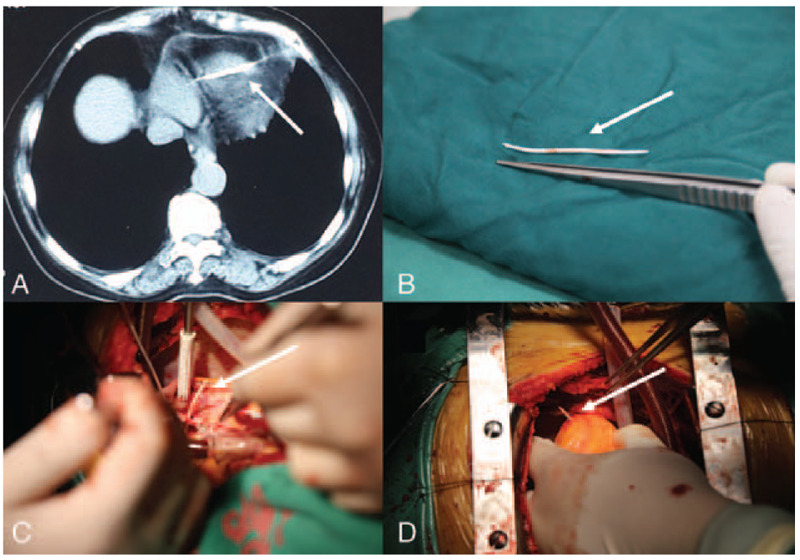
(A) Chest computed tomography showing a high-density shadow extending from the bottom of the heart to the anterior chest wall (indicated by arrow). (B) Foreign body after removal with forceps for scale. (C) Foreign body piercing the posterior flap of the tricuspid valve (indicated by the arrows). (D) Foreign body penetrating the anterior wall of the right ventricle (indicated by the arrows).
